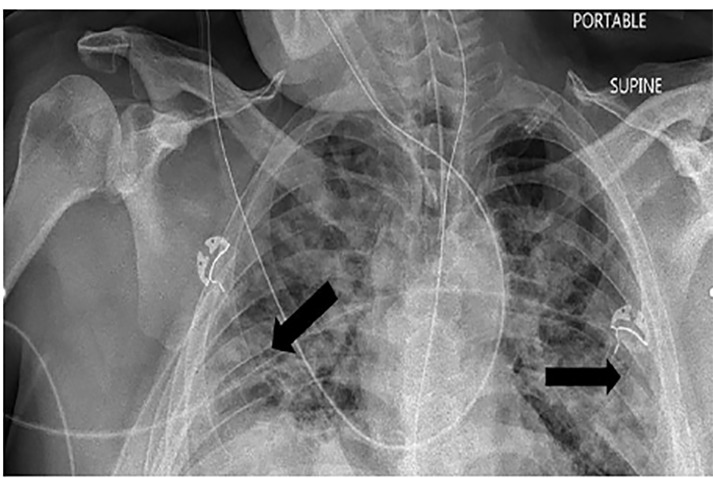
Chest radiograph with peripheral airspace opacities (arrows).
